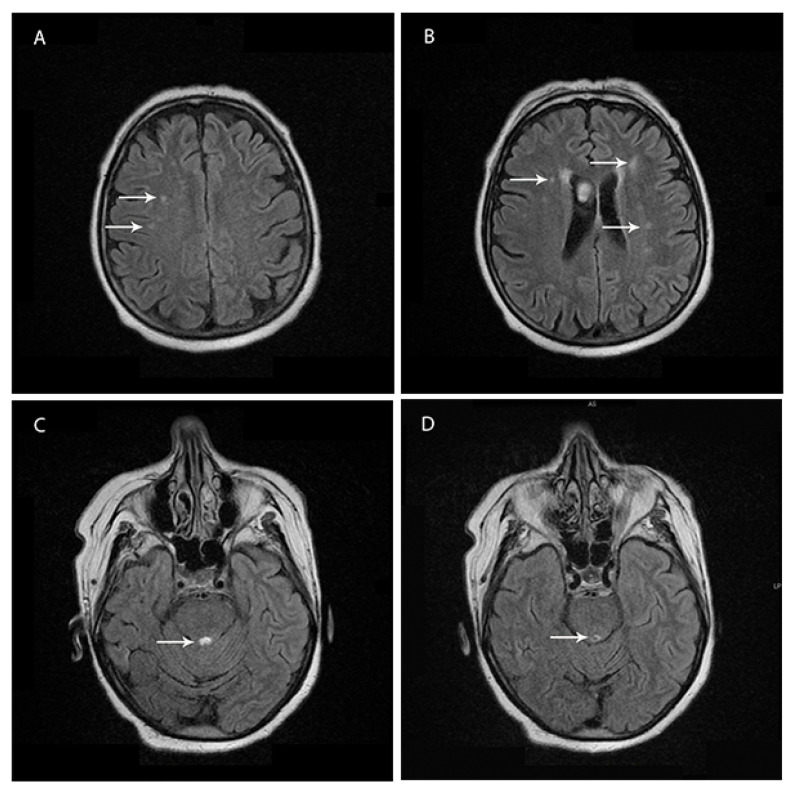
(A–D) Brain magnetic resonance imaging (MRI) study on T2-fluid attenuation-recovery (FLAIR). (A,B) Bilateral supratentorial subcortical increased signal intensities on FLAIR images. (C,D) Periaqueductal grey matter hyperintensity in FLAIR images.
